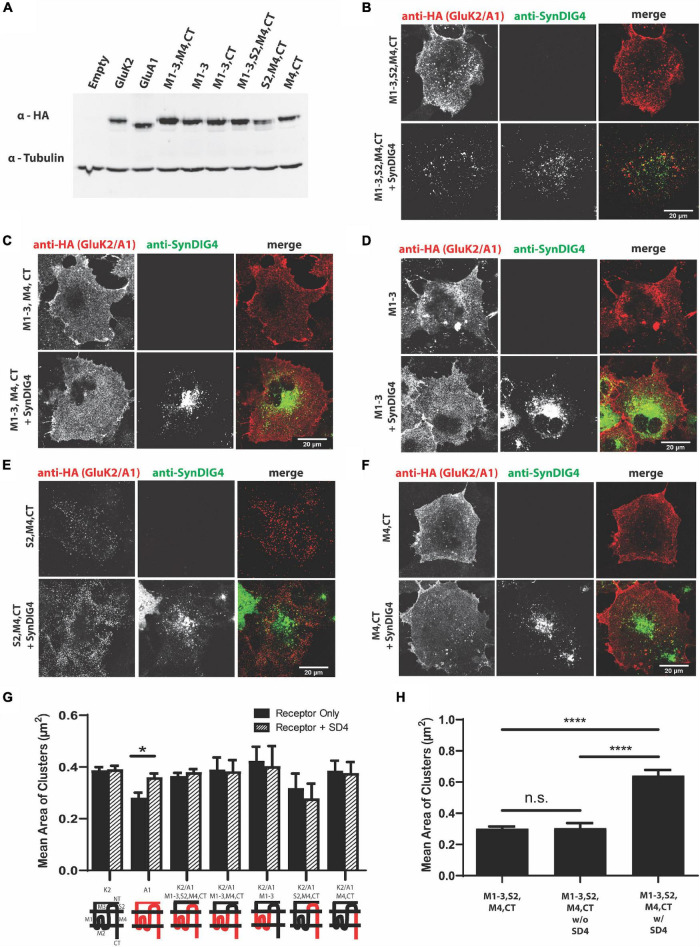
The N-terminus of GluA1 is dispensable for clustering with SD4. (A) Immunoblot depicting expression of GluK2/GluA1 chimeras. Homologous domains of GluA1 were inserted into the backbone of GluK2 (as shown in Table 2 of Methods). β-tubulin was used as the loading control. (B–F) Representative confocal images of COS cells transfected with either a GluK2/A1 chimeric receptor alone, or co-transfected with SD4. Scale bar = 20 μm. (G) Graph depicts the mean area of clusters when GluK2, GluA1, and each GluK2/A1 chimera is expressed either alone or co-expressed with SD4. (H) Graph depicts the mean cluster size of M1-3, S2, M4, CT chimera stratified for either co-localized or not co-localized with SD4. M1-3, S2, M4 alone (*n* = 11), M1-3, S2, M4 w/o SD4 (*n* = 13), M1-3, S2, M4 w/SD4 (*n* = 13). Data are represented as mean ± SEM; n.s. not significant; p* < 0.05; *p* < 0.0001; one-way ANOVA with *post-hoc* Tukey’s test.
